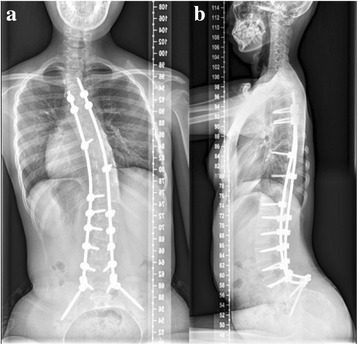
Anterior-posterior and lateral X-ray at 12-month follow-up. a Anterior-posterior X-ray showed the Cobb of scoliosis (T5-L2) is 29°. b Lateral X-ray showed Cobb of thoracolumbar lordosis (T9-S1) is 70°
